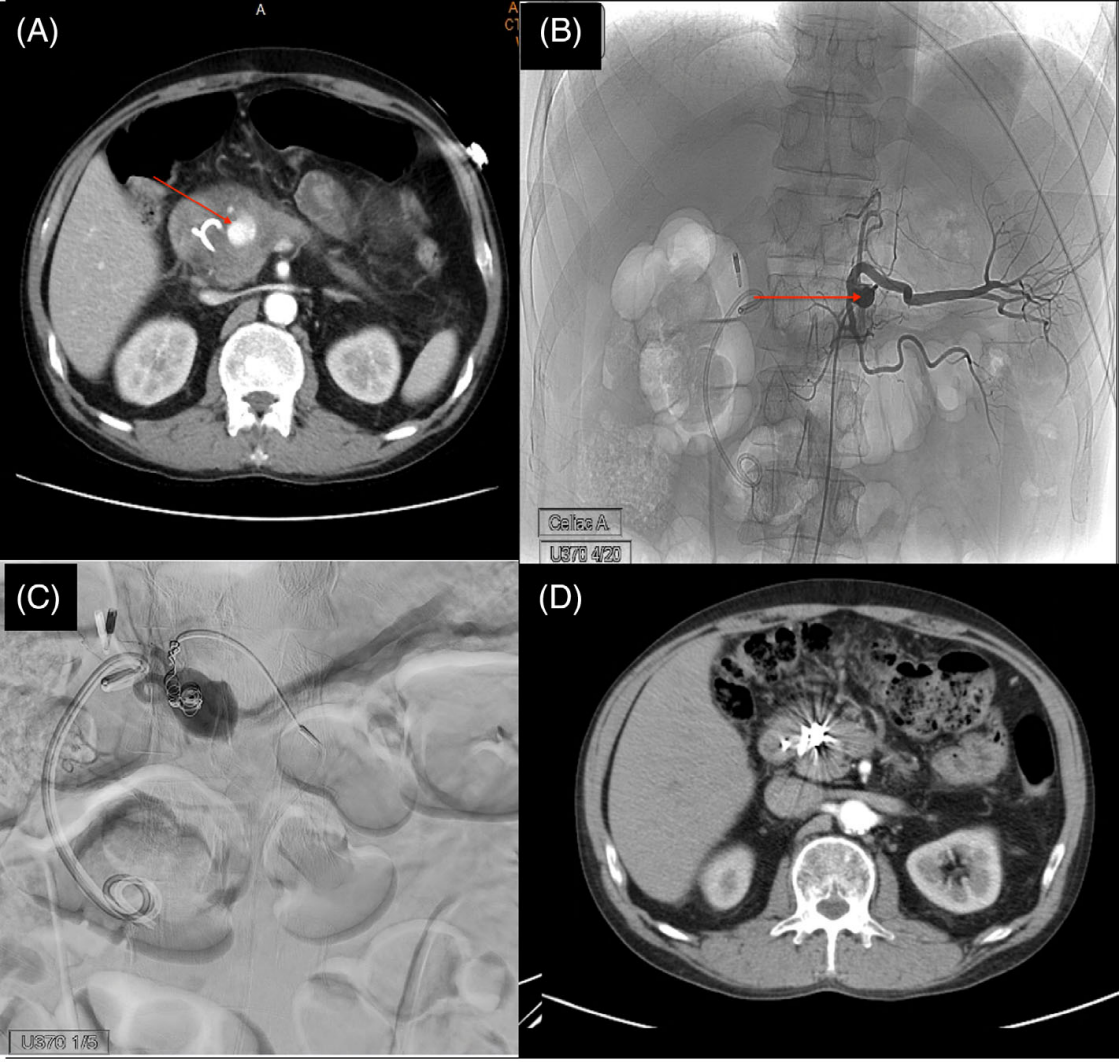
Contrast enhanced abdominal computed tomography (CT) scan showed pseudoaneurysm (arrow) originating from the gastroduodenal artery (A). Angiogram showed a compatible result with CT scan (B). Angiographic embolization with combination of coils and gelfoam was introduced to gastroduodenal artery (C). Followed CT scan showed successful coil embolization of the pseudoaneurysm and reduction in the size of the pseudocyst at pancreatic head (D).
